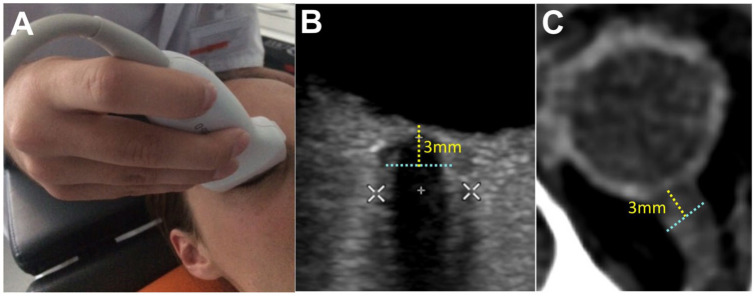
Standardized Measurement of the Optic Nerve Sheath Diameter (ONSD): (a) application of the high-frequency linear probe for transorbital sonography, (b) ultrasound image demonstrating the measurement of the internal ONSD (ONSDint, light blue dashed line) 3 mm posterior to the globe. The white calipers indicate the measurement of the external ONSD, and (c) corresponding ONSD measurement on an axial CT scan.
